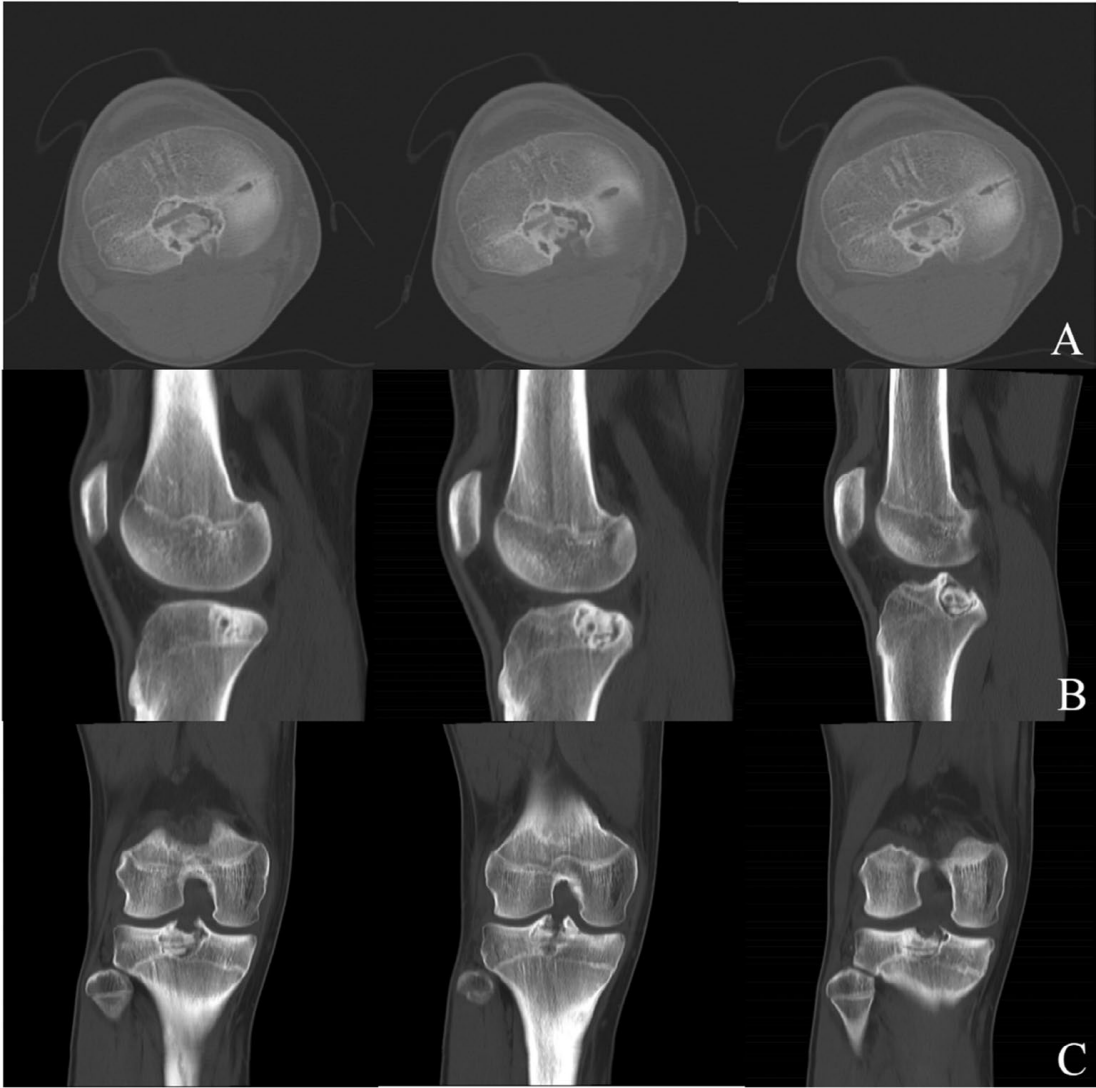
CT scan showing at 12 months of follow‐up of a good osteointegration of the bone graft: axial view (A), sagittal view (B), and coronal view (C).
